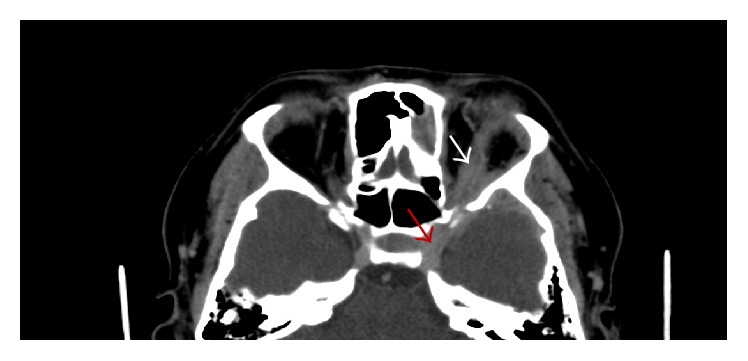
CT with contrast in the sinuses demonstrates left intraorbital involvement (white arrow) that extends through the cavernous sinus determining pachymeningeal enhancement (red arrow).
